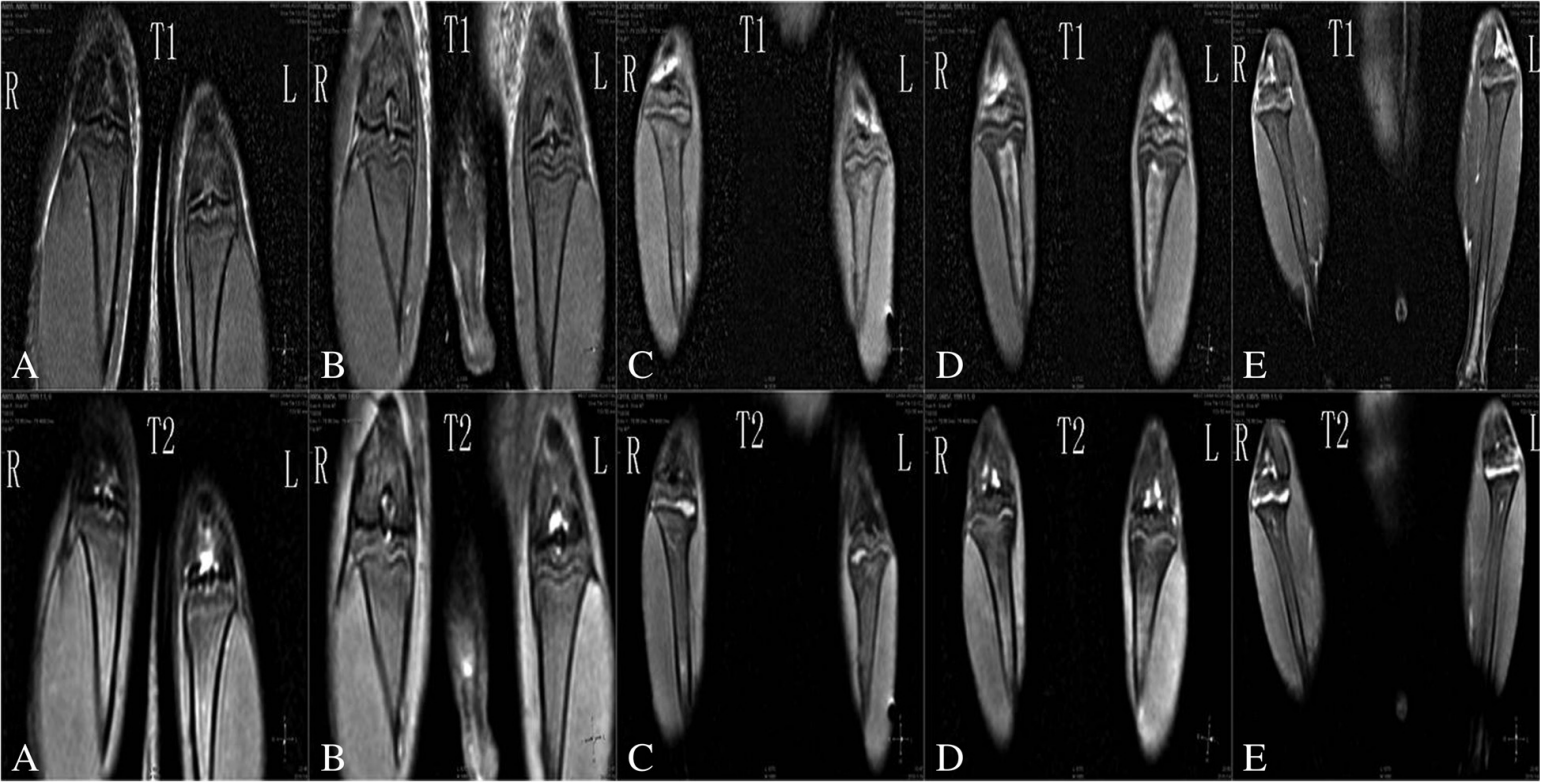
MR images of the epiphyseal plate at 12 weeks. (A) Epiphyseal plate *shows narrow, discontinuous and rough; the intensity shows low and uneven*. (B) Epiphyseal plate *shows narrow, discontinuous and rough; the intensity shows low and uneven*. (C) Epiphyseal plate *shows narrow, discontinuous and rough; the intensity shows higher or low and uneven*. (D) Epiphyseal plate *shows narrow, discontinuous and rough; the intensity shows low and uniformity*. (E) Epiphyseal plate *shows narrow, discontinuous and rough; the intensity shows higher or low and uneven*.
